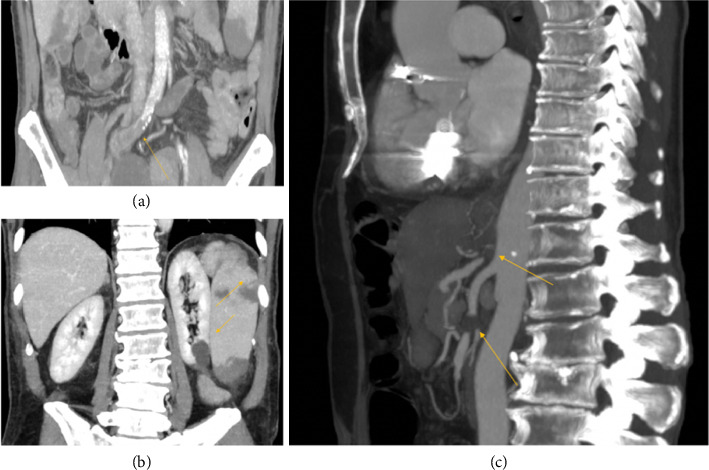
Computed tomography findings. (a) Occlusive thrombus of the external iliac artery (arrow). (b) Thrombotic occlusions of the left kidney and spleen (arrows). (c) Occlusive thrombus in the proximal superior mesenteric artery and at the origin of the celiac trunk (arrows).
